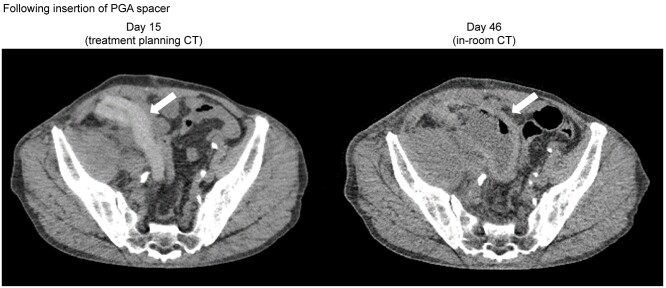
The treatment planning CT image and in-room CT image (46 days after the insertion of PGA spacer) of Case 2. Arrow: PGA spacer.
